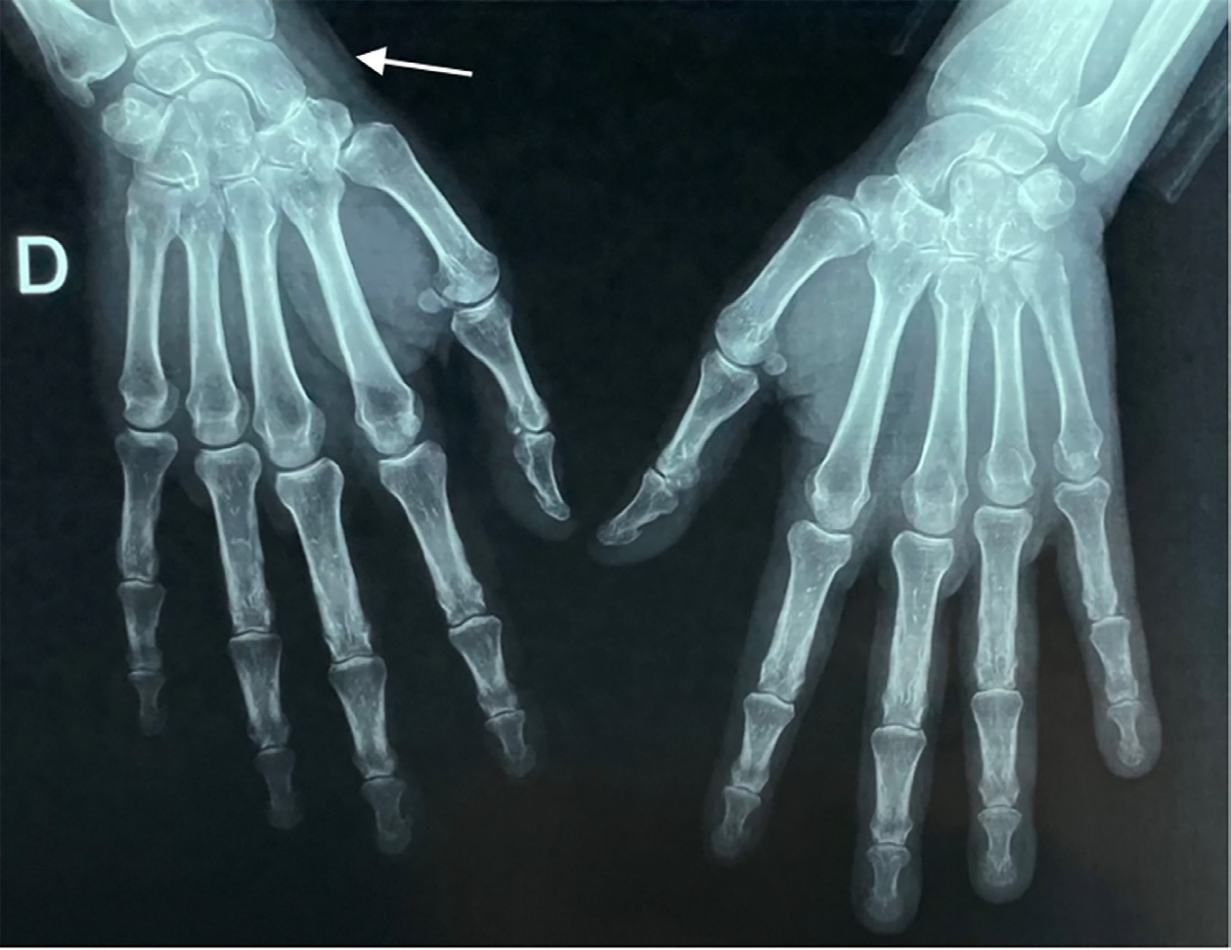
X-ray of the front of the hands and wrists showing thickening of the soft tissues of the right wrist (arrow).
